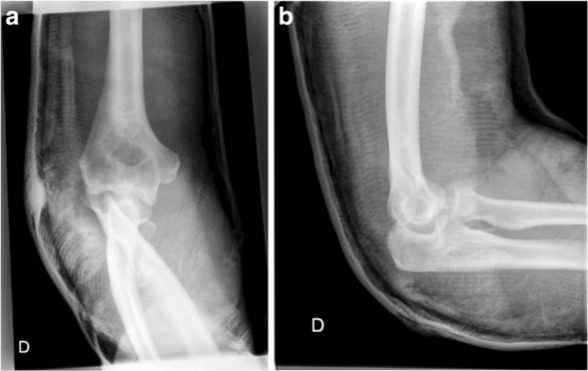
Postreduction radiographs (anteroposterior (a) and lateral (b) views) show an ulnohumeral joint partially reduced, a comminuted radial head fracture and a fracture of the coronoid process
